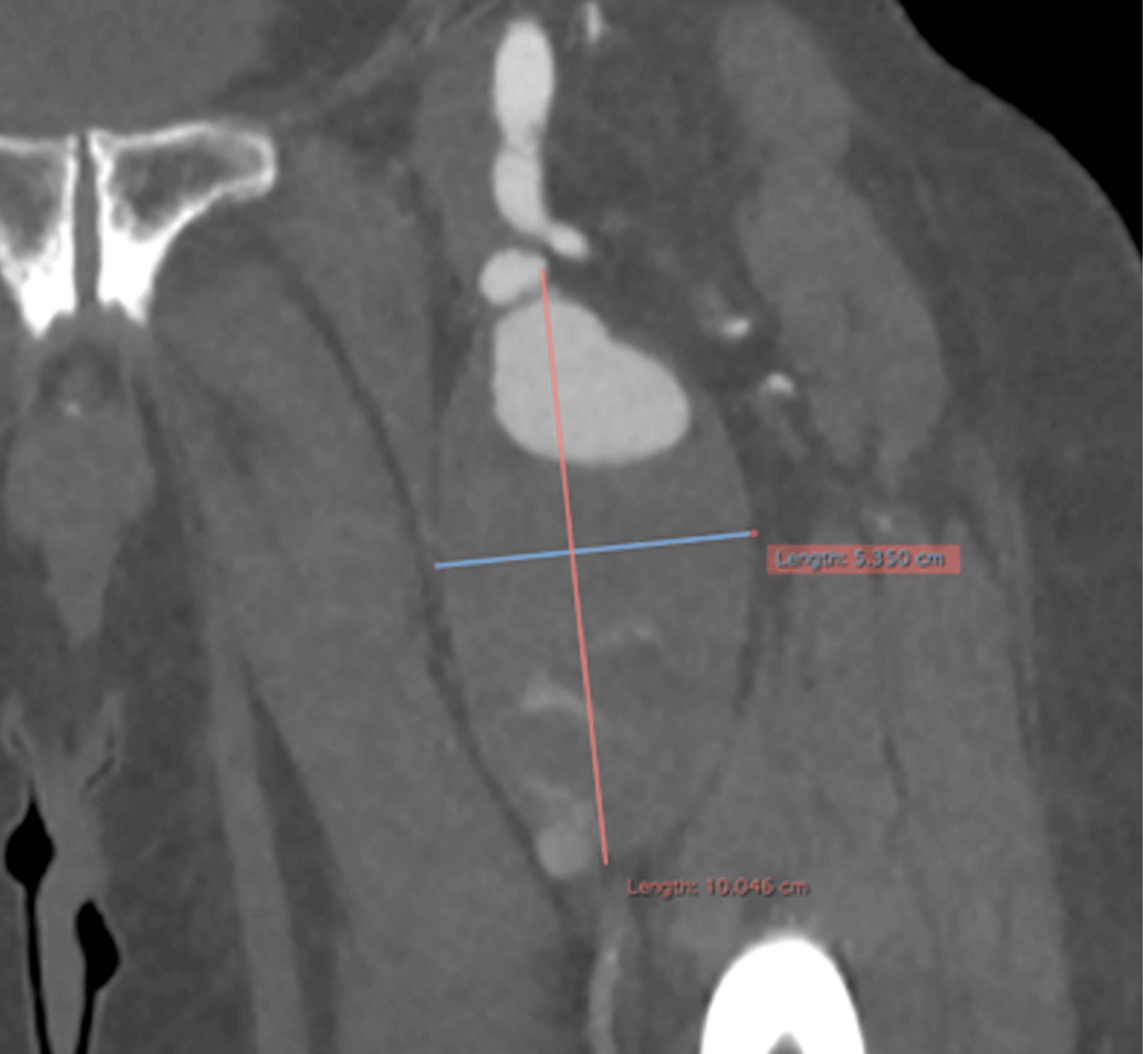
CT angiography, frontal plane It shows the left deep femoral artery aneurysm with its craniocaudal and transverse extension and irregular mural thrombus.
